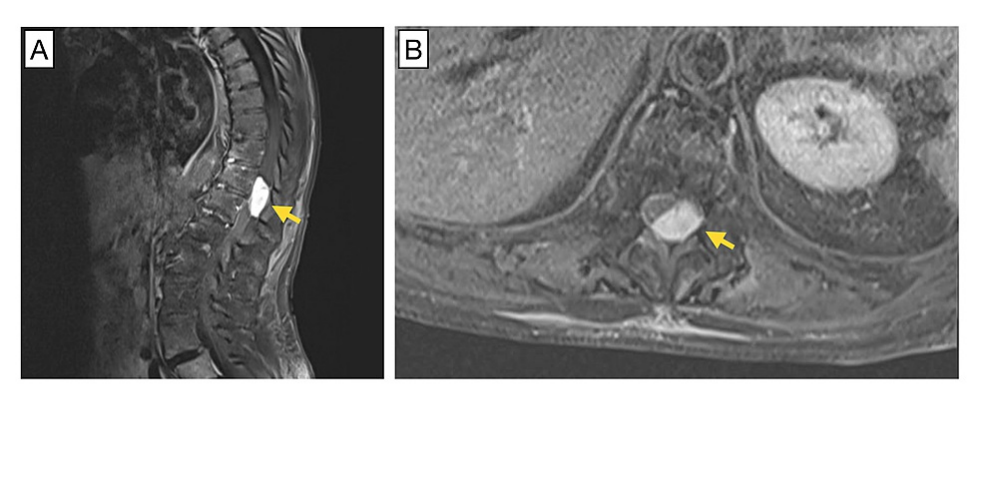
MRI of the spinal column prior to surgical resection. Sagittal (A) and transverse (B) pre-operative MRI demonstrate a contrast-enhancing 3.1-cm T11-12 left lateral mass with intradural, extramedullary localization (yellow arrows).
